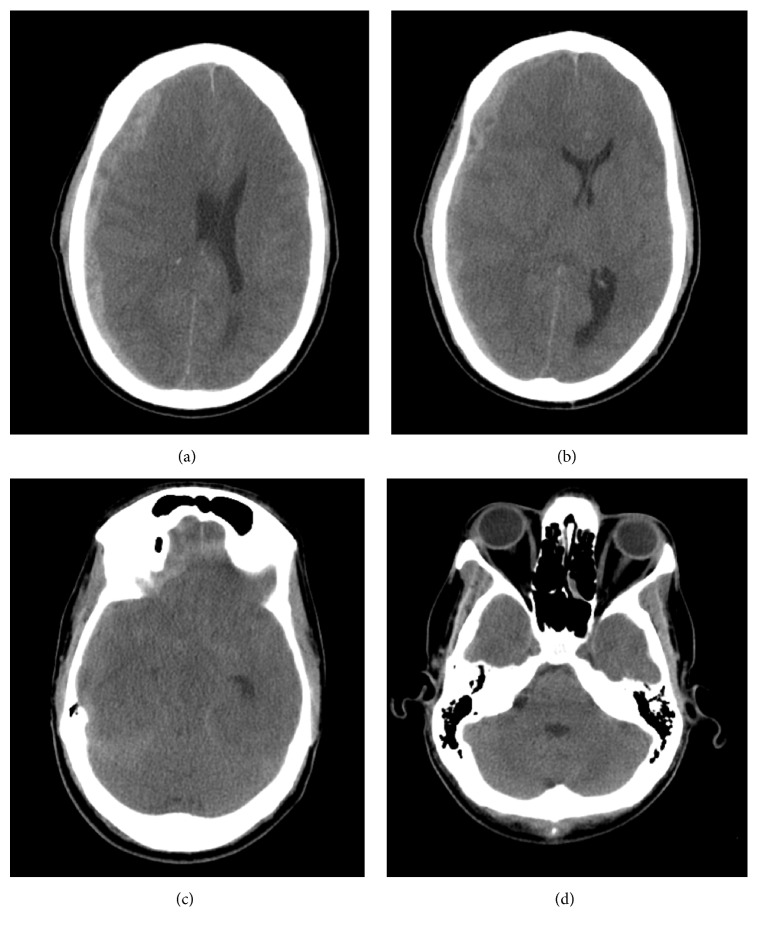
Initial CT scan showing panhemispheric, mixed density subdural mass with significant mass effect ((a) and (b)). There is significant effacement of the right temporal horn and basilar cisterns consistent with herniation (c), though the 4th ventricle is still patent (d).
